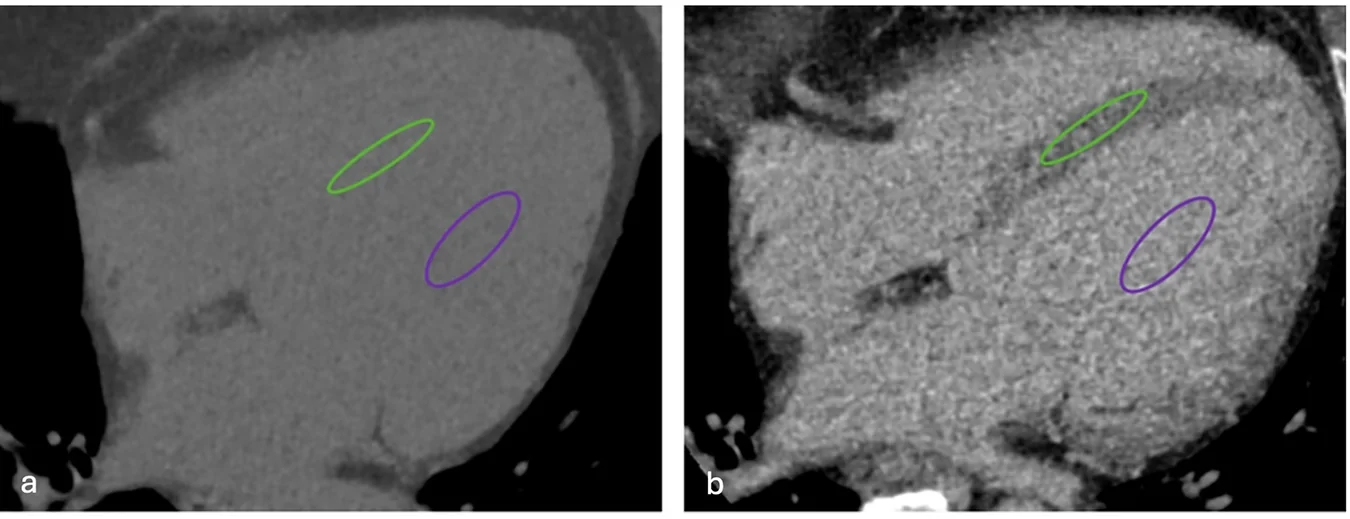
Example of myocardial extracellular volume (ECV) quantification on delayed-phase cardiac CT. Circular regions of interest (ROIs) are placed in the mid-ventricular septal myocardium and in the left ventricular blood pool on delayed contrast CT images. These ROIs are propagated to corresponding pre- (a) and post-contrast (b) scans to measure attenuation (Hounsfield units, HU)
